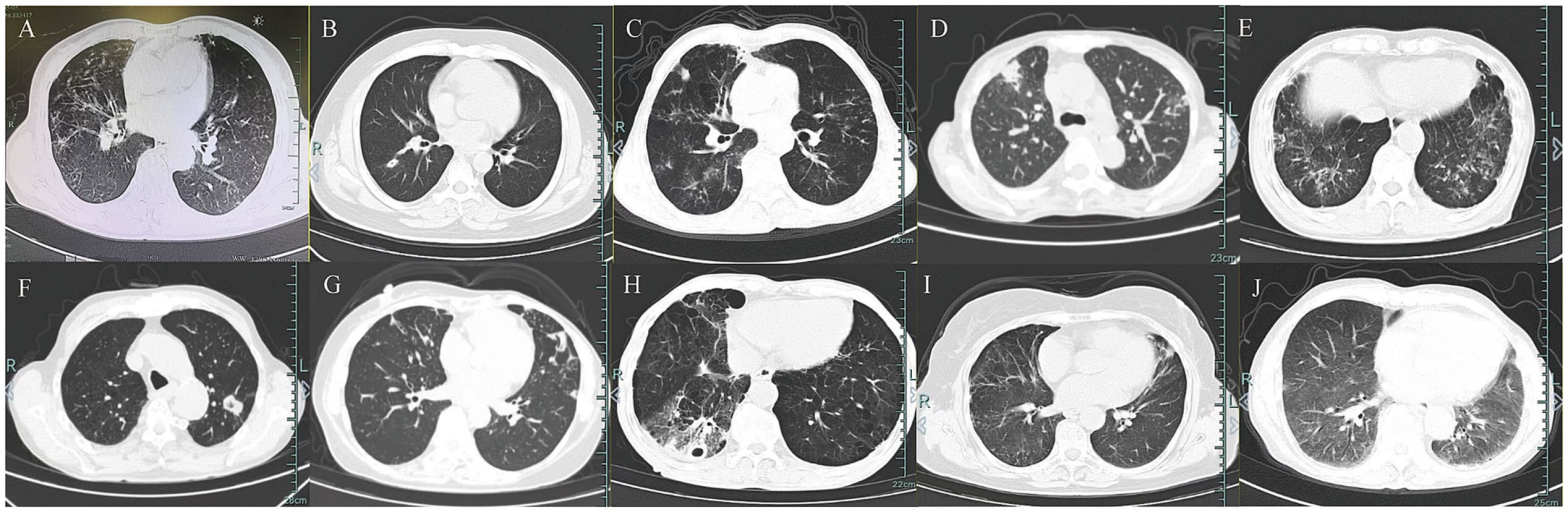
Chest imaging features in 10 patients. (A–G) Chest CT in patients with *T. marneffei* infection. (H–J) Chest CT in patients with non-*T. marneffei* infection.
